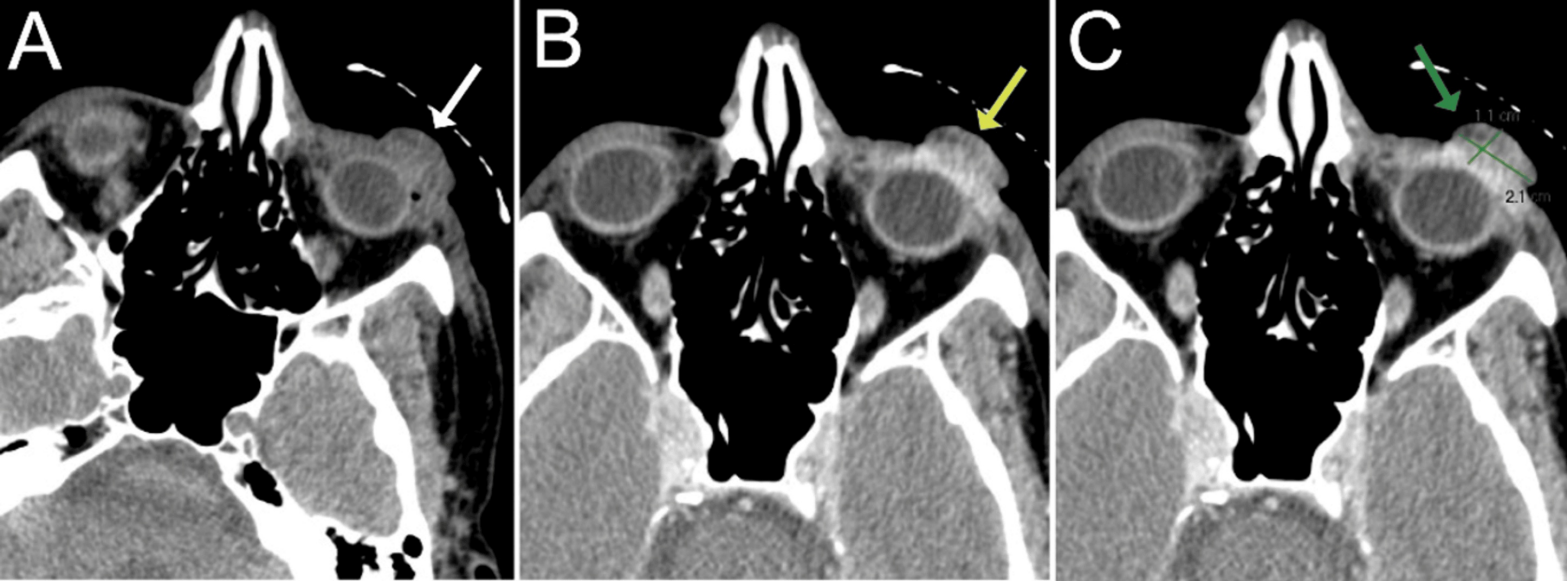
Axial orbital computed tomography scans. (A) Pre-contrast and (B) post-contrast scans reveal an exophytic-enhancing conjunctival lesion around the inferior aspect of the anterior left globe measuring approximately 1.1 cm (anterior-posterior) × 2.1 cm (transverse) × 1.7 cm (craniocaudal). There was no involvement of the eyelid, lacrimal gland, extraocular muscles, or left lens.
